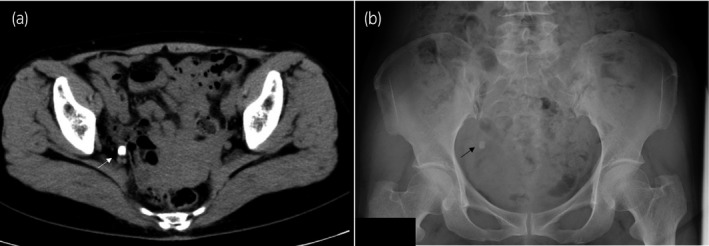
A 7 × 7 mm stone at the right distal ureter detected on plain CT (a; arrowhead) and plain abdominal radiography (b; arrowhead).
